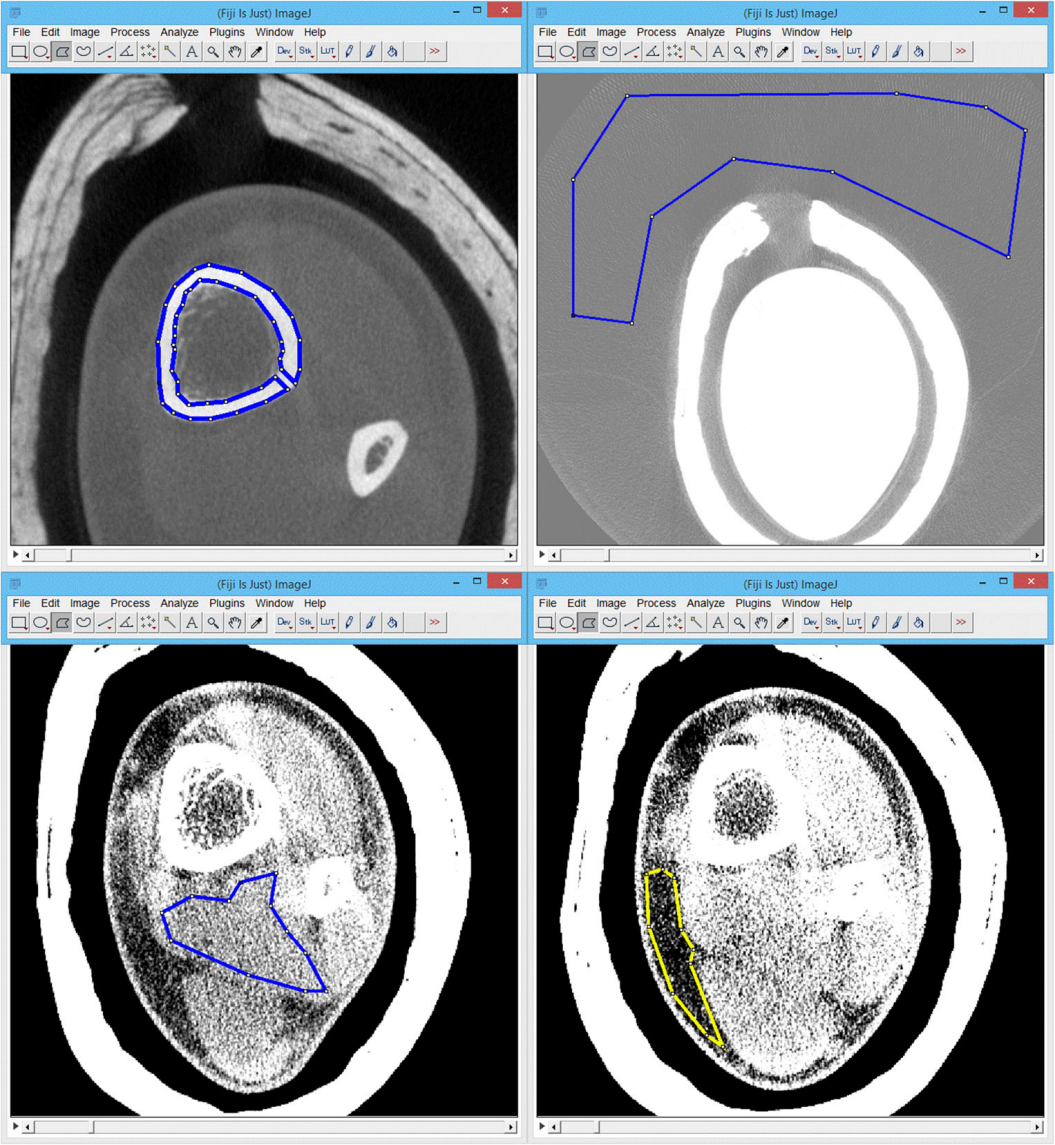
The procedure of ROI measurements utilizing a polygonal selection tool in FIJI shown for cortical bone (top left), air (top right), muscle (lower left), and fat (lower right) in a sample CBCT of an ankle
